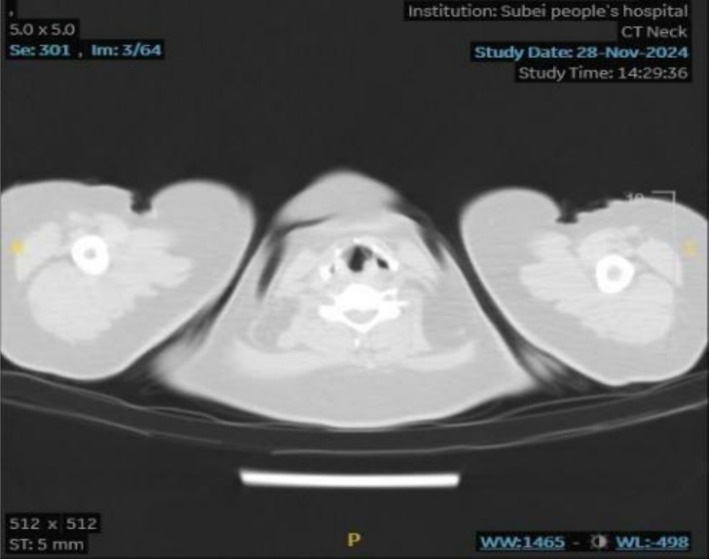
Preoperative neck CT scan.
